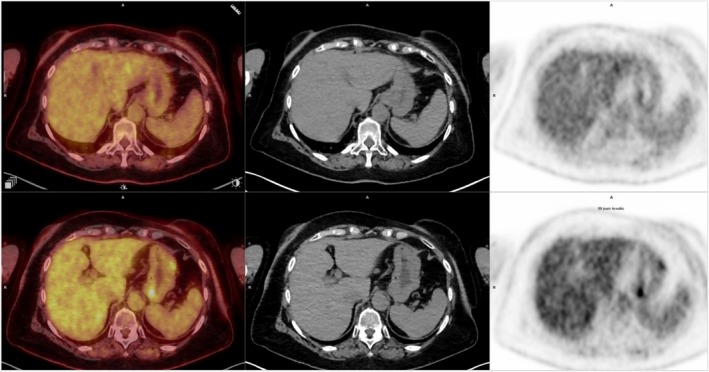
Comparison of FDG PET/CT images taken in April 2024 (top) and October 2023 (bottom), on axial fused PET/CT slice (left, middle) and axial PET slice (right), showing a partial response of the cardia lesion and a complete responses of other gastric lesions, with disappearance of the focal uptake in the antral‐fundic region.
